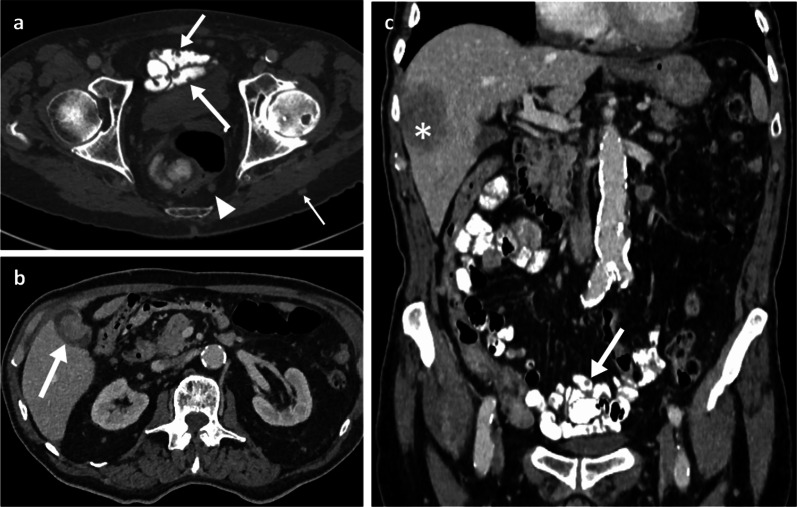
Oral and intravenous portal phase contrast-enhanced CT axial (a, b) and coronal (c) of an 85-year-old male patient with numerous small melanoma metastases throughout the bowel with nodular mucosal thickening (arrows in a, c), metastatic peritoneal nodules (arrowhead in a), gallbladder metastasis (arrow in b), liver metastasis (* in c) and subcutaneous metastasis (thin arrow in a)
